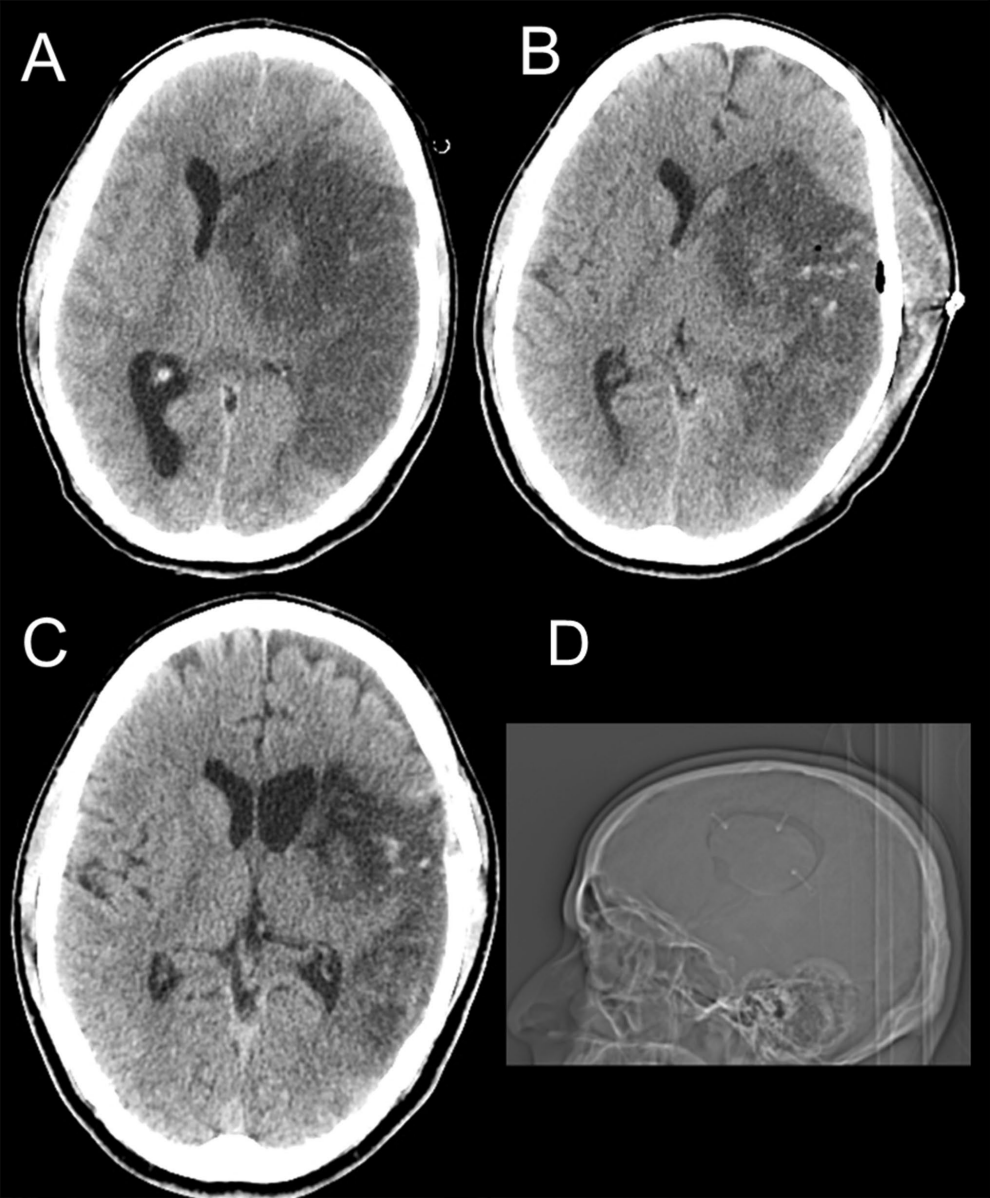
A 36-year-old male with malignant L MCA stroke. a Preoperative axial CT scan demonstrating severe midline shift. b 48-h postoperative axial CT scan with debulking of ischemic tissue from the left temporal lobe, there is evident midline shift despite clinical improvement in neurological status. c An axial CT head of the same patient at 1 month following surgery. d This is a skull scout image, showing the craniotomy size, the free riding mini-plates attached to the craniotomy flap only and that the bone did not sink into the cranial vault 3 years post-surgery. This patient recovered to mRS 2
